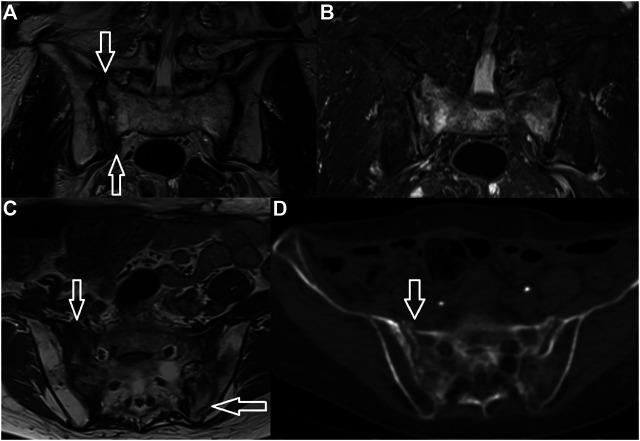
Insufficiency fracture. H-shaped sacral fracture is well-visible both on MRI and CT (arrows) (A–TSE T2 WI, B–TSE T2 FAT SAT, C–TSE T1 WI, D–CT).
